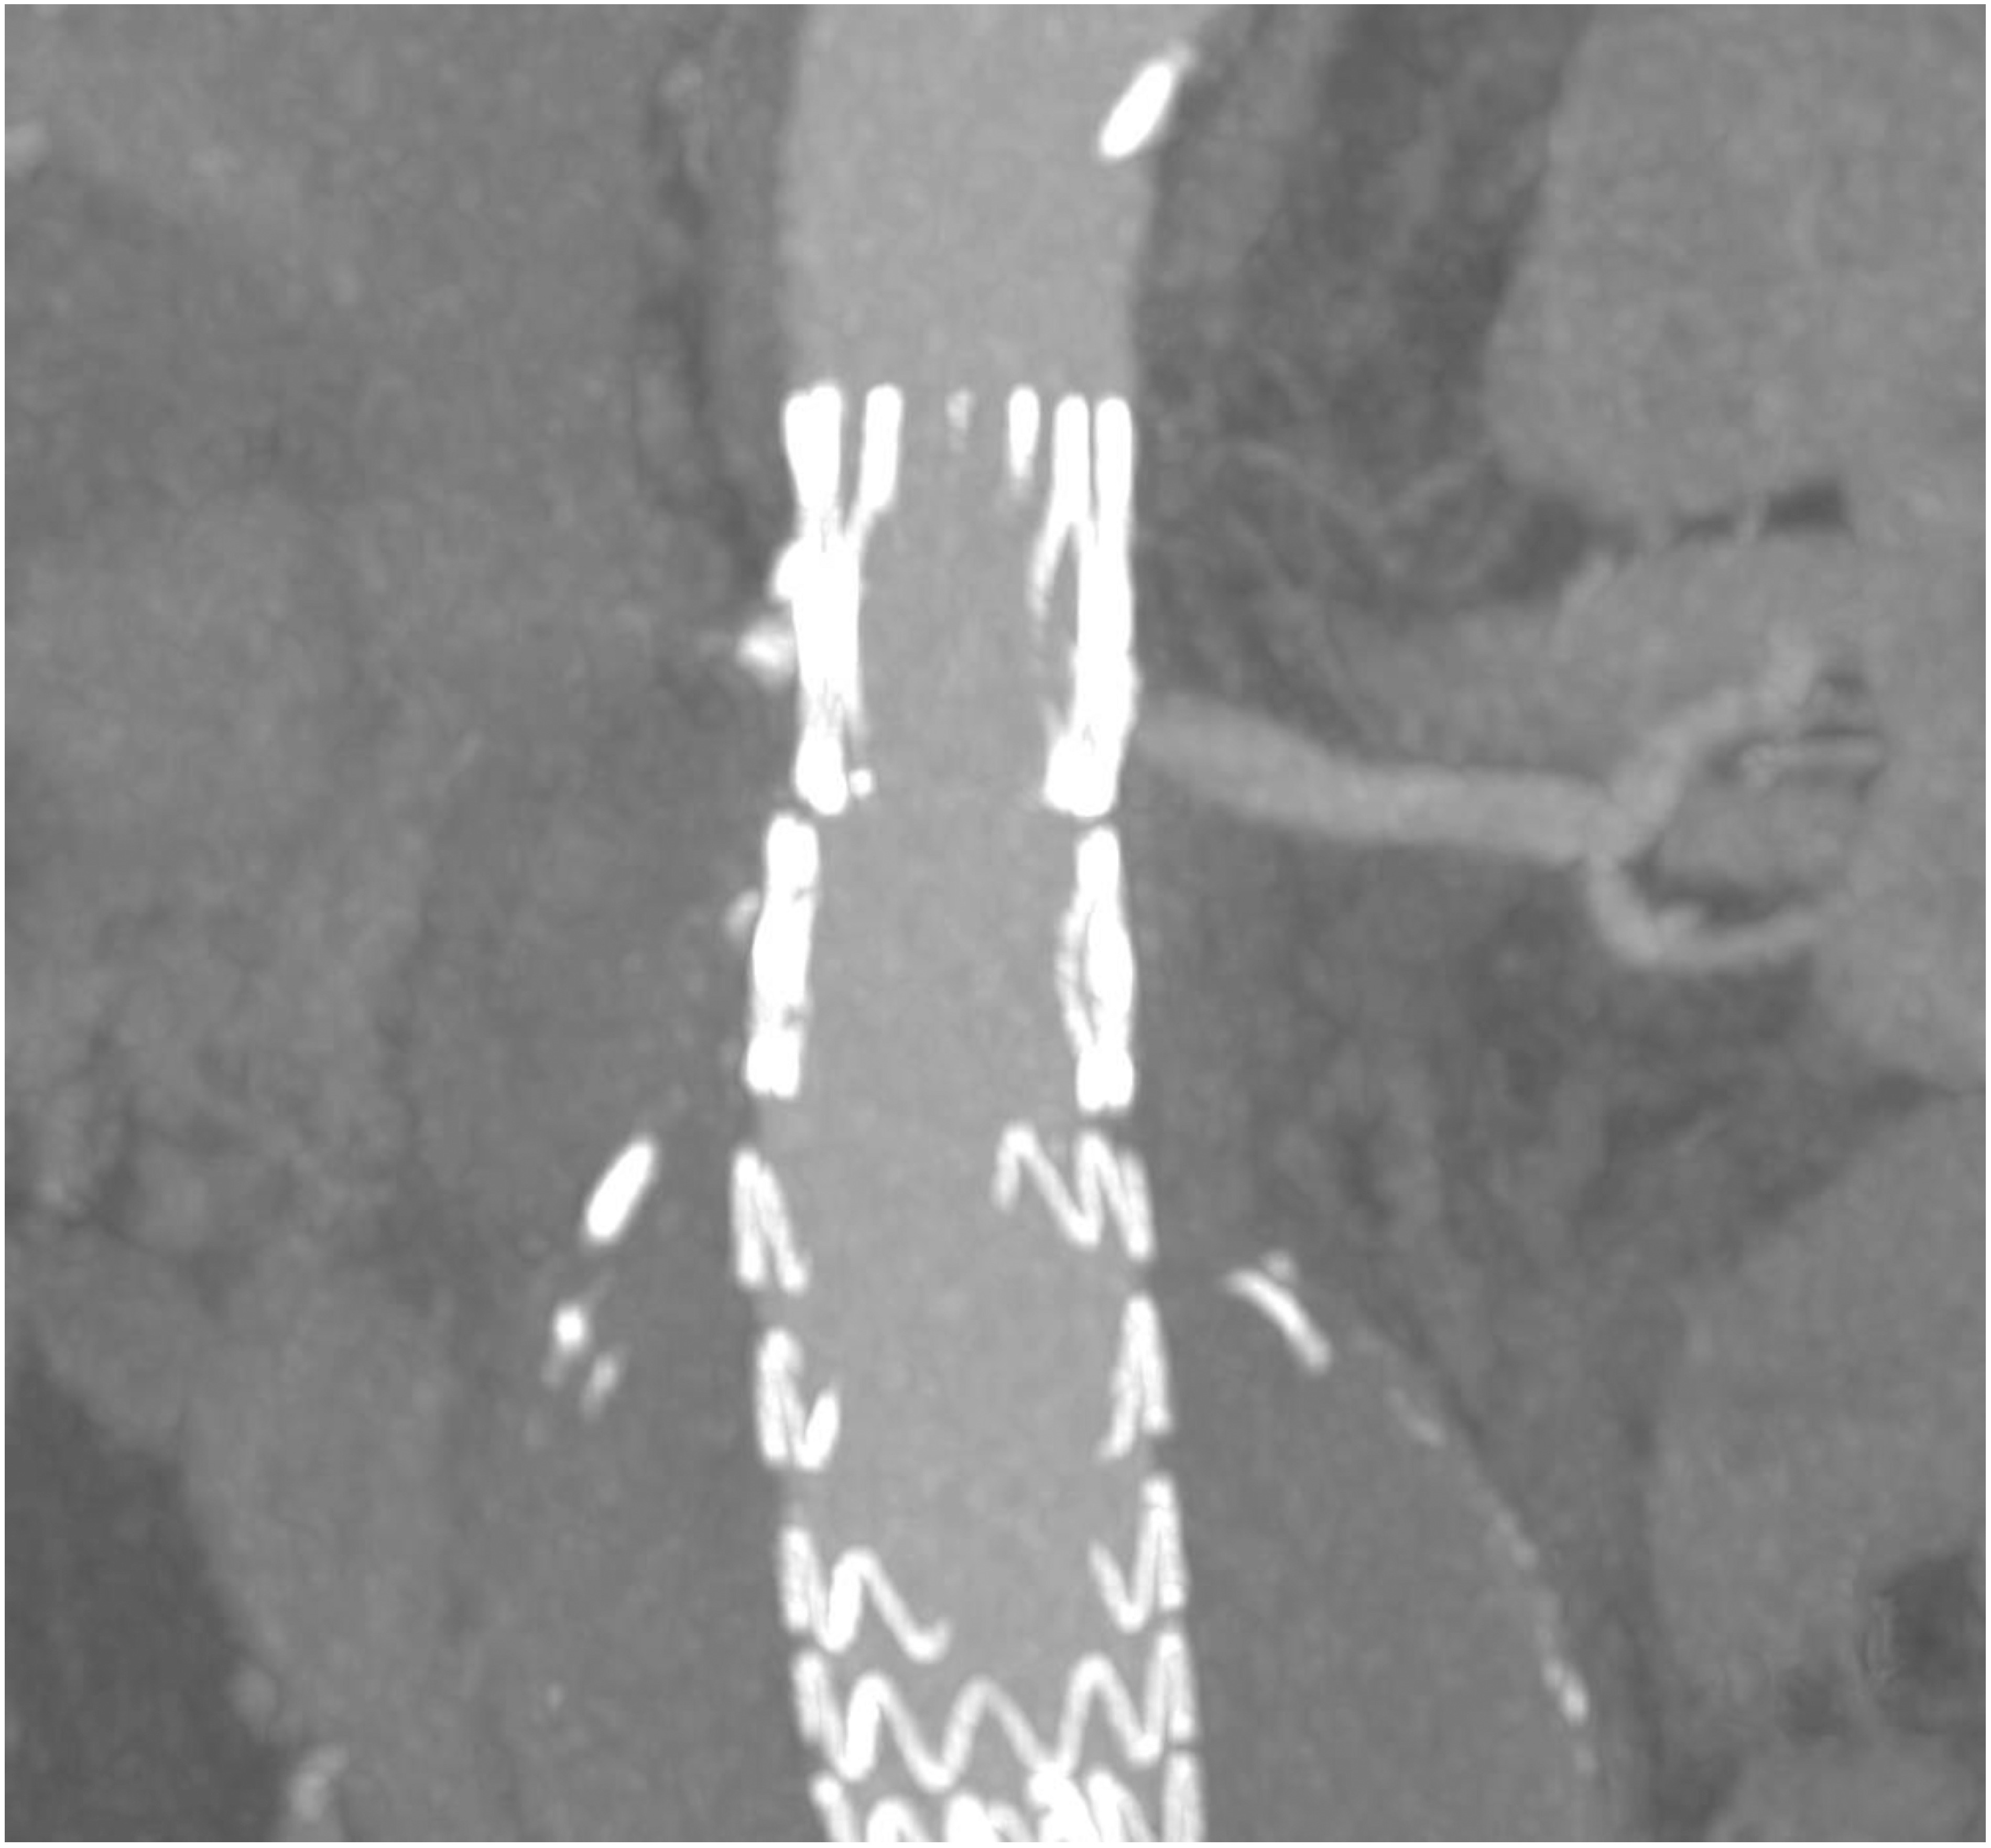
Fig. 3 Postoperative computed tomography angiography image demonstrating the resolution of the refractory type 1a endoleak following wrap technique.
